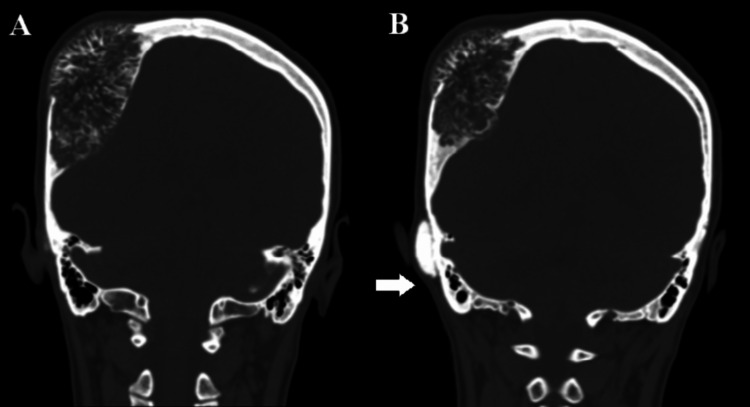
Brain CT scan bone window (A) Coronal view showing right parietal sunburst appearance of a bone tumor: a radiographic pattern that happens when the rate of lesion growth exceeds the periosteum capacity to lay down Sharpey’s fibers (collagen fibers that tie periosteum down to the bone). This results in splaying these fibers in radiating fashion. (B) Another coronal view showing the right parietal bone tumor and another expansile bone tumor arising from the outer cortex of the right mastoid part of the temporal bone (marked by a white arrow).
